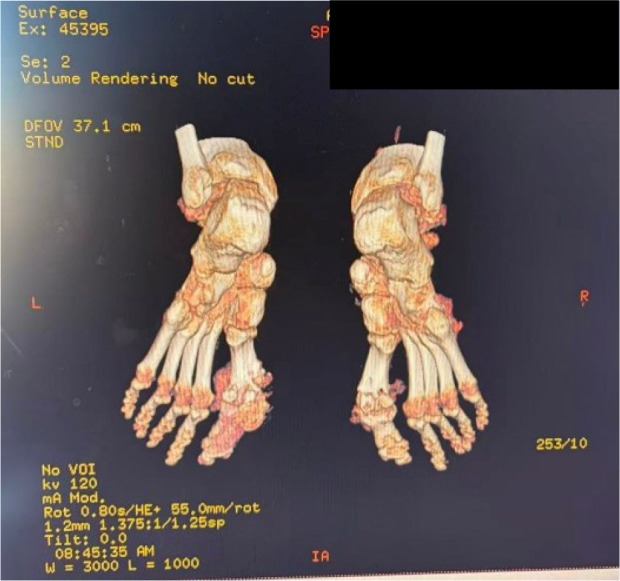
CT scans of the patient’s bilateral feet.
